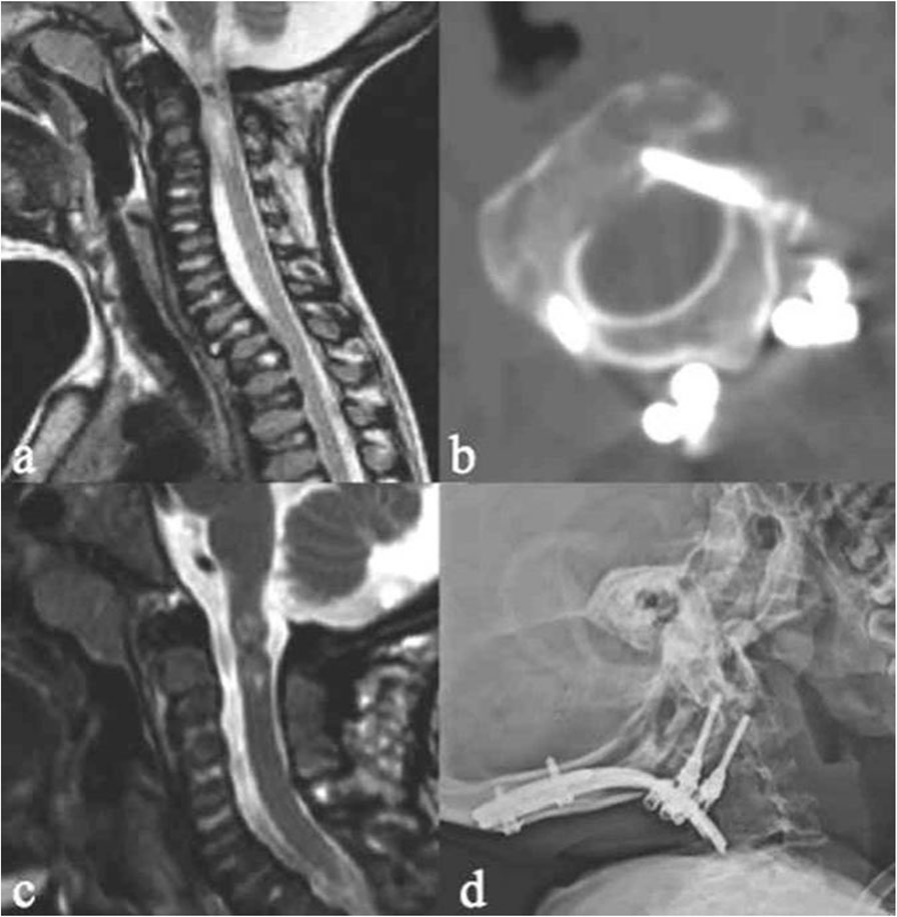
a MRI showing severe post-traumatic myelopathy after a minor backwards fall in a previously simply decompressed MPS IV child. b C2 pars screws, c postoperative MRI, and d plain x-rays at 4 years follow-up
